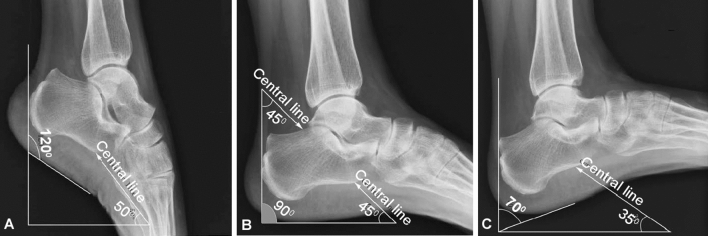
Calcaneal radiography in different positions in a male volunteer (28 years of age). (A) When the ankle was at 30° dorsiflexion and the tube tilts up to 50° cephalad, the tarsus and talus cannot be clearly demonstrated because they are overlapped with the subtalar joint and the sustentaculum tali. The calcaneus is elongated. (B) When the ankle was at the neutral location (0° dorsiflexion) and the tube tilts 45° cephalad, the tarsus, talus, subtalar joint, and sustentaculum tali can all be clearly shown. The calcaneal body is demonstrated in normal ratio with normal width and length. (C) When the ankle was at 20° plantarflexion and the tube tilts 35°, the tarsus, talus, subtalar joint, and sustentaculum tali can all be clearly displayed. The width and length of the calcaneus are normal.
